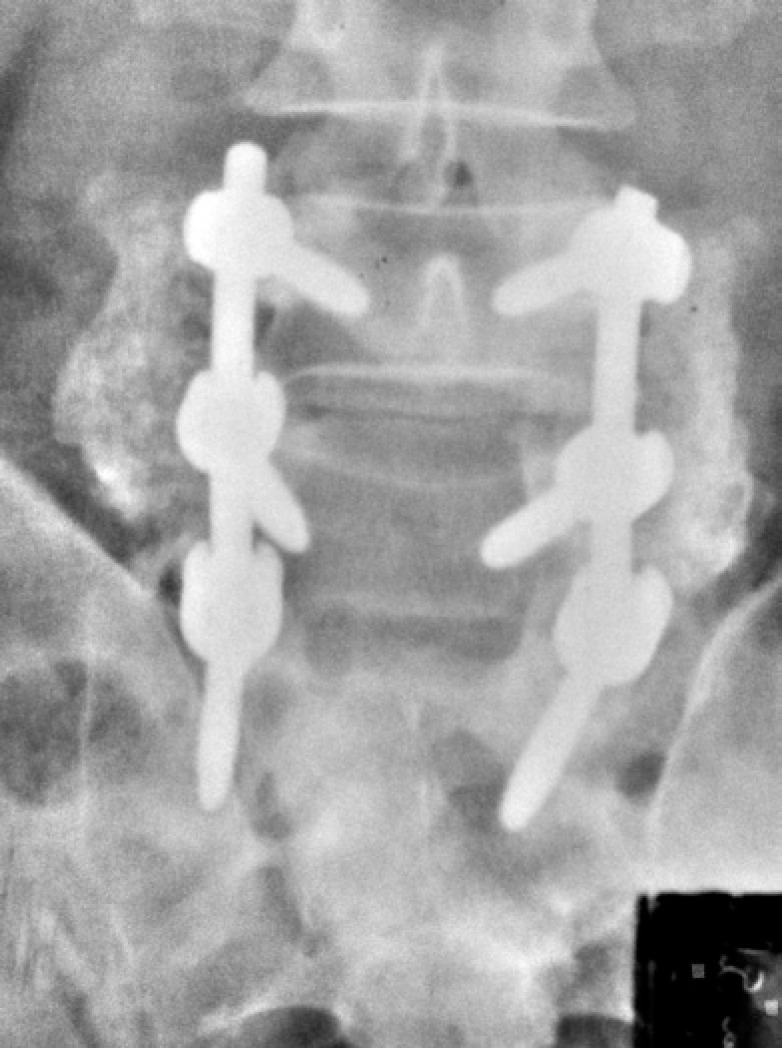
Anteroposterior radiography of the lumbosacral spine showing complete posterolateral fusion
